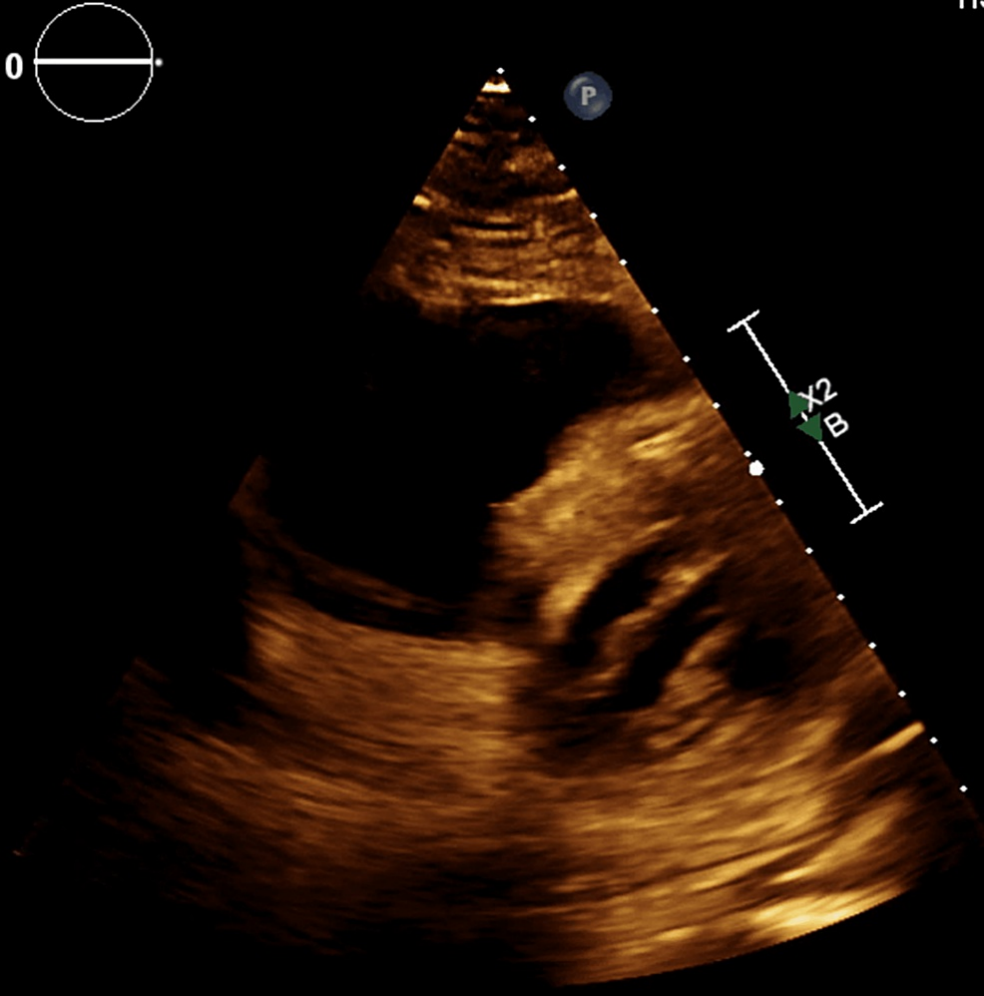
Echocardiogram showing dilated right ventricle.
